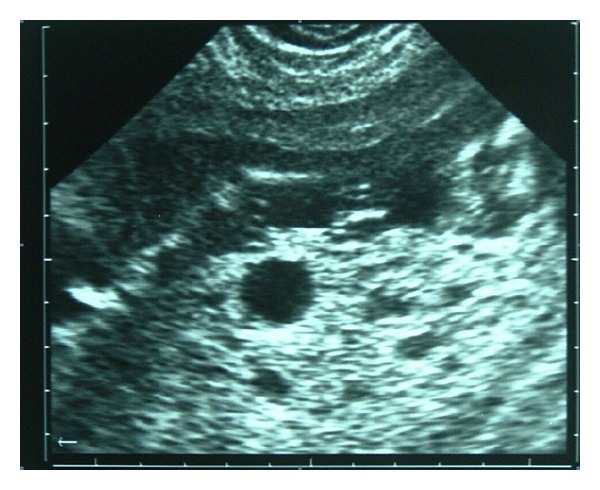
Ultrasound: Placentomegaly with multiple large anechoic areas. Is the entity partial mole?
